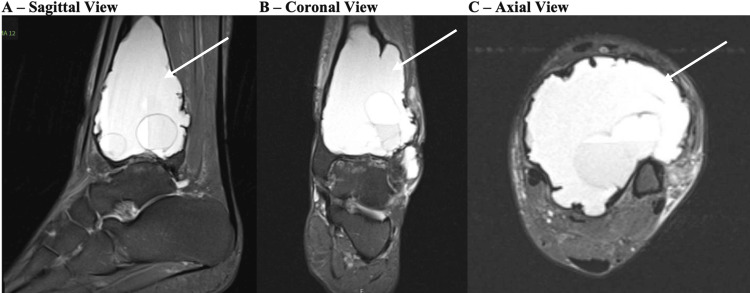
Repeat MRI Sagittal (A), coronal (B), and axial (C) MRI views of the left ankle of the patient when she returned to the clinic several months later. The lesion is marked with arrows.
